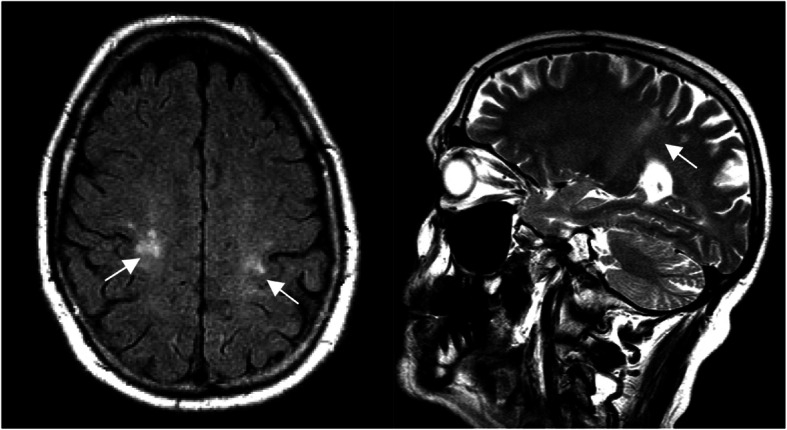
Pattern B2: MR image of an adult patient. Relatively isolated signal changes of the pyramidal tract (arrows, T2w sagittal left, axial Flair right). The cerebellum shows no signal changes. Patient 38 (onset: 60 years, age at MRI: 69 years).
